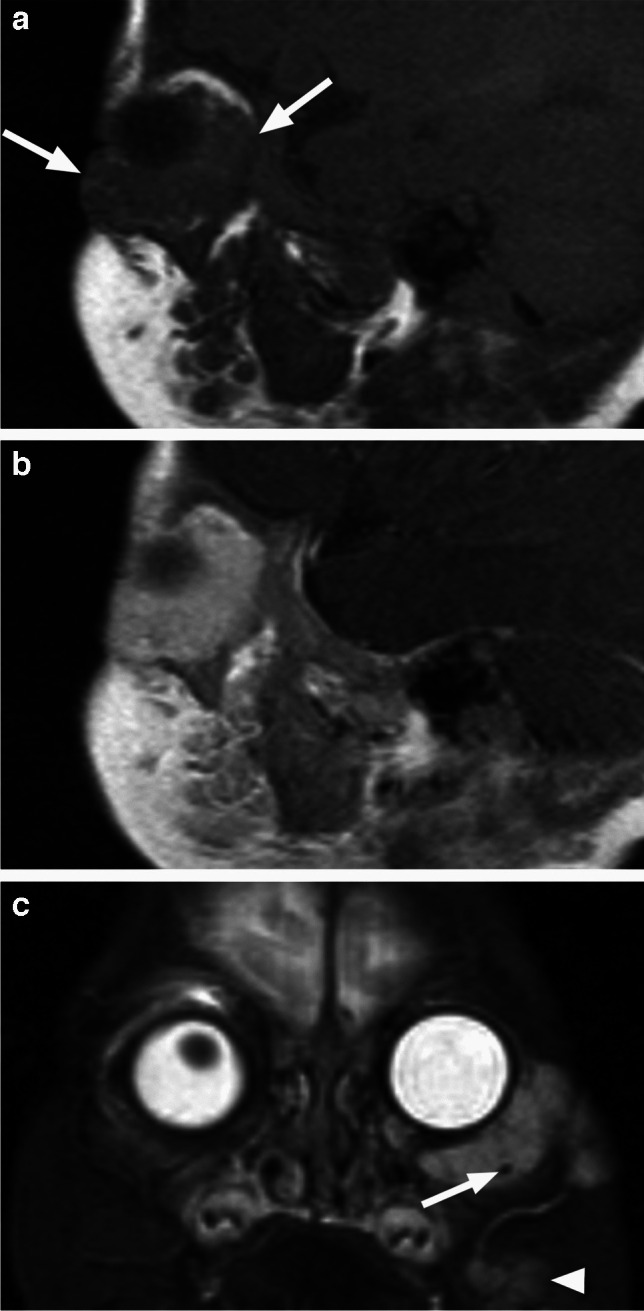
Venous malformation. Sagittal T1-weighted image (a) shows an isointense preseptal and postseptal intraorbital lesion (arrows), which is enhancing on the corresponding delayed postcontrast image (b). Coronal STIR image (c) demonstrates increased signal of the lobulated mass with an internal oval hypointensity, indicative of a phlebolith (arrow), without flow voids. The irregular trans-spatial lesion is contiguous with an additional facial component (arrowhead), which is also present on the sagittal images
